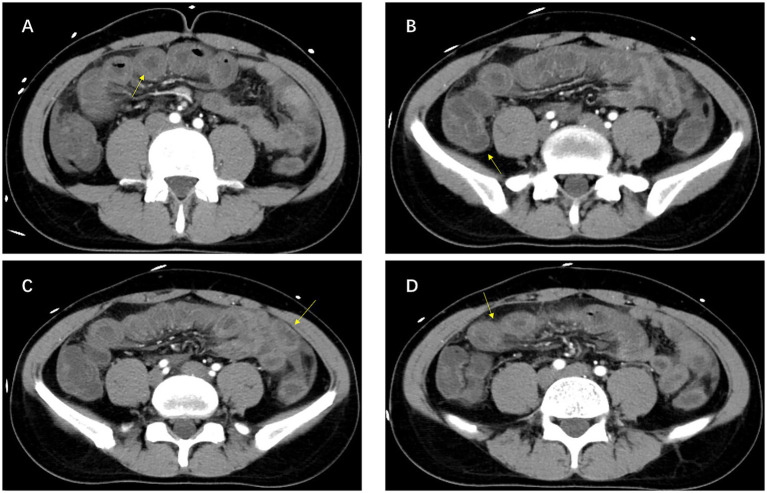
Contrast-enhanced abdominal CT findings during an acute abdominal attack. (A–D) Axial contrast-enhanced CT images demonstrate segmental ileal wall thickening with submucosal edema (arrows), accompanied by a small amount of free intraperitoneal fluid.
